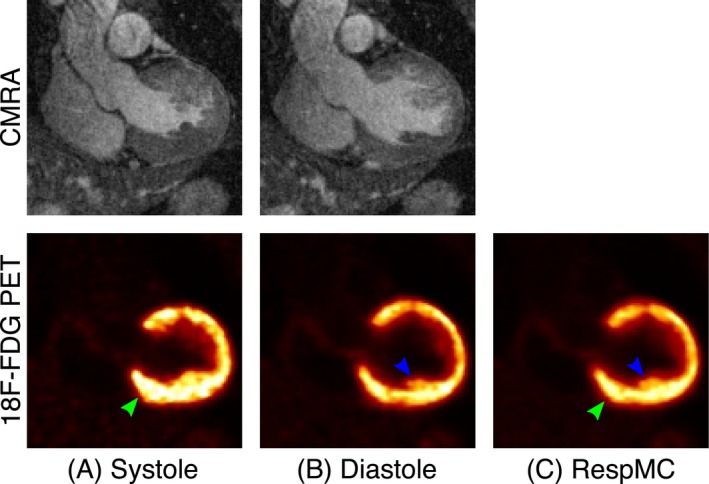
Coronal views from CAD patient 3 data set showing respiratory motion‐corrected (A) systolic and (B) diastolic CMRA and ^18^F‐FDG PET images alongside the (C) respiratory motion‐corrected (RespMC) PET image. RespMC aggregates data acquired at different cardiac phases, resulting in cardiac‐induced blurring of small structures (blue arrow) and apparent thickening of the myocardium (green arrow)
